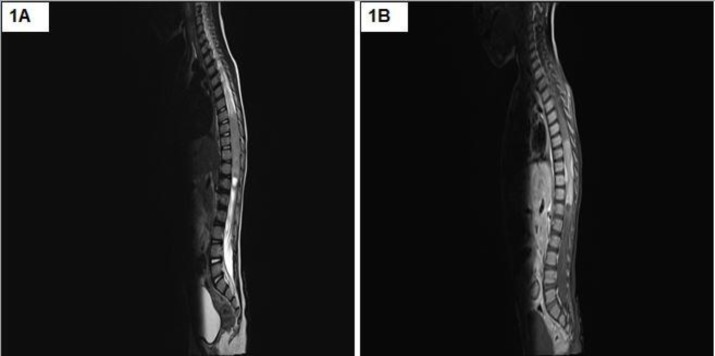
MRI showing an intramedullary mass lesion extending from D8-L1 which was hypointense on T1 (1A) and hyperintense on T2 (1B) with a cyst at lower pole
